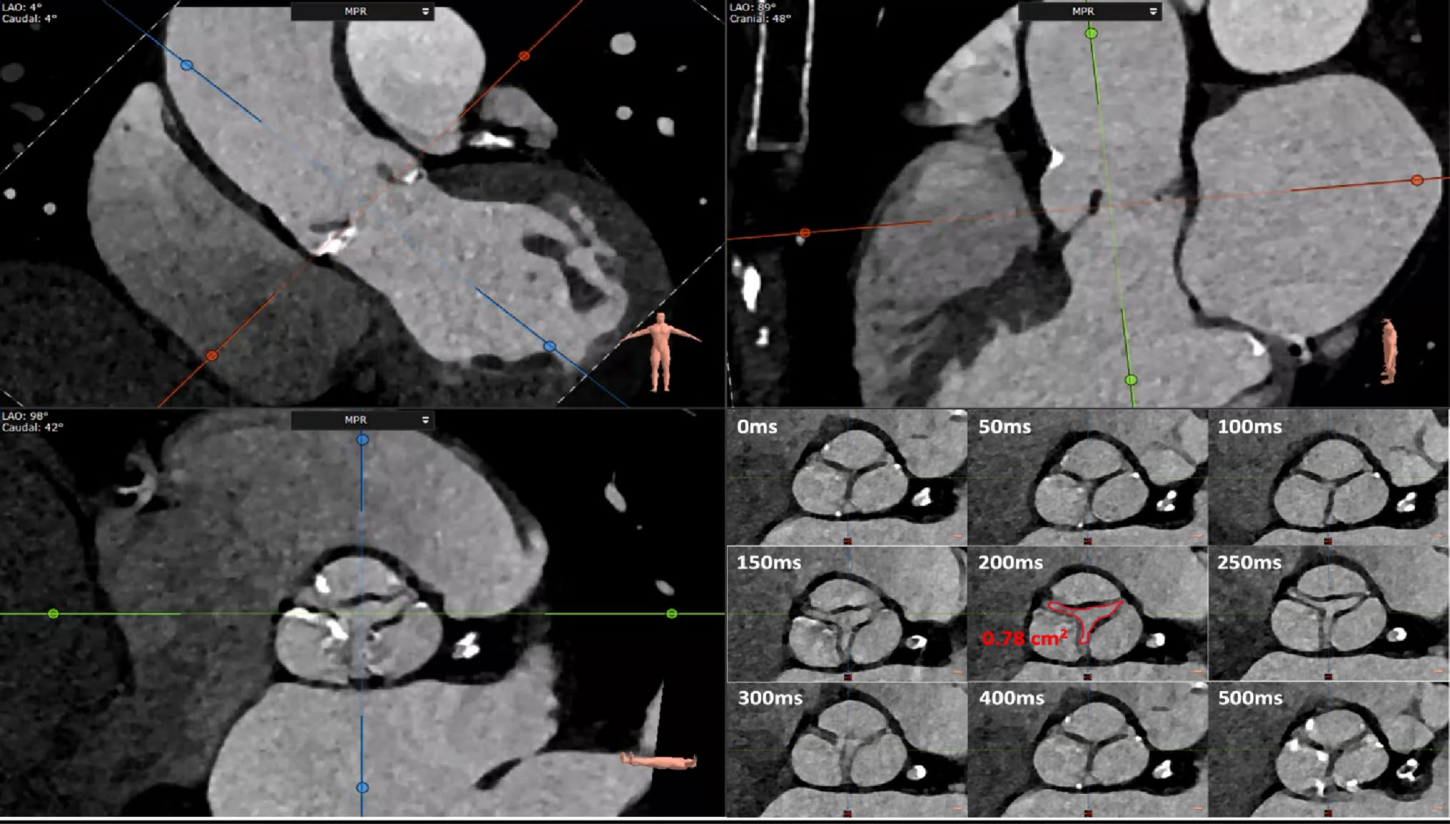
Computed tomography valve analysis. Representative images of multiplanar reconstruction (top left and top right) and visualization of the aortic valve orifice (bottom left). Midsystolic planimetry of the aortic valve area is shown in the bottom right image. White numbers indicate the time (in milliseconds) between the R-wave and image acquisition. The red area represents the midsystolic aortic valve area at 200 ms
